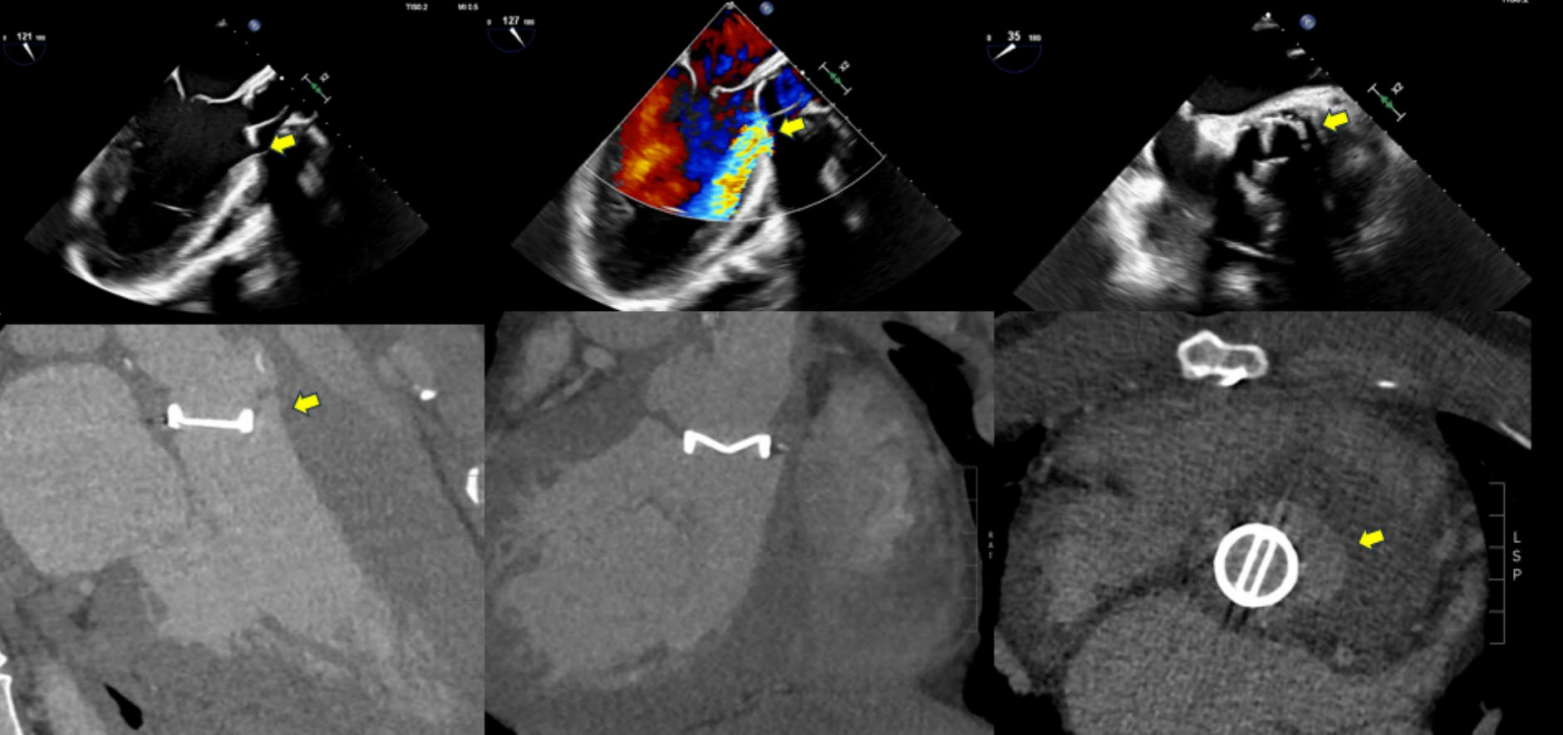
Images of a 44-year-old gentleman with a history of valve-sparing aortic root replacement for an aneurysmal aortic root approximately 9 years ago, followed by mechanical aortic valve replacement (AVR) 5 years later. He presented with heart failure, and his transthoracic echocardiogram revealed severe paravalvular aortic regurgitation. Transesophageal echocardiogram confirmed severe paravalvular regurgitation and valve dehiscence. A subsequent CT coronary angiogram confirmed valve dehiscence and an aortic root abscess which was culture negative. *Top panel:* transesophageal echocardiogram images depicting valve dehiscence and severe paravalvular aortic regurgitation (arrow). *Bottom panel:* CT demonstrating valve dehiscence and the aortic root abscess (arrow). The patient was referred for surgery
